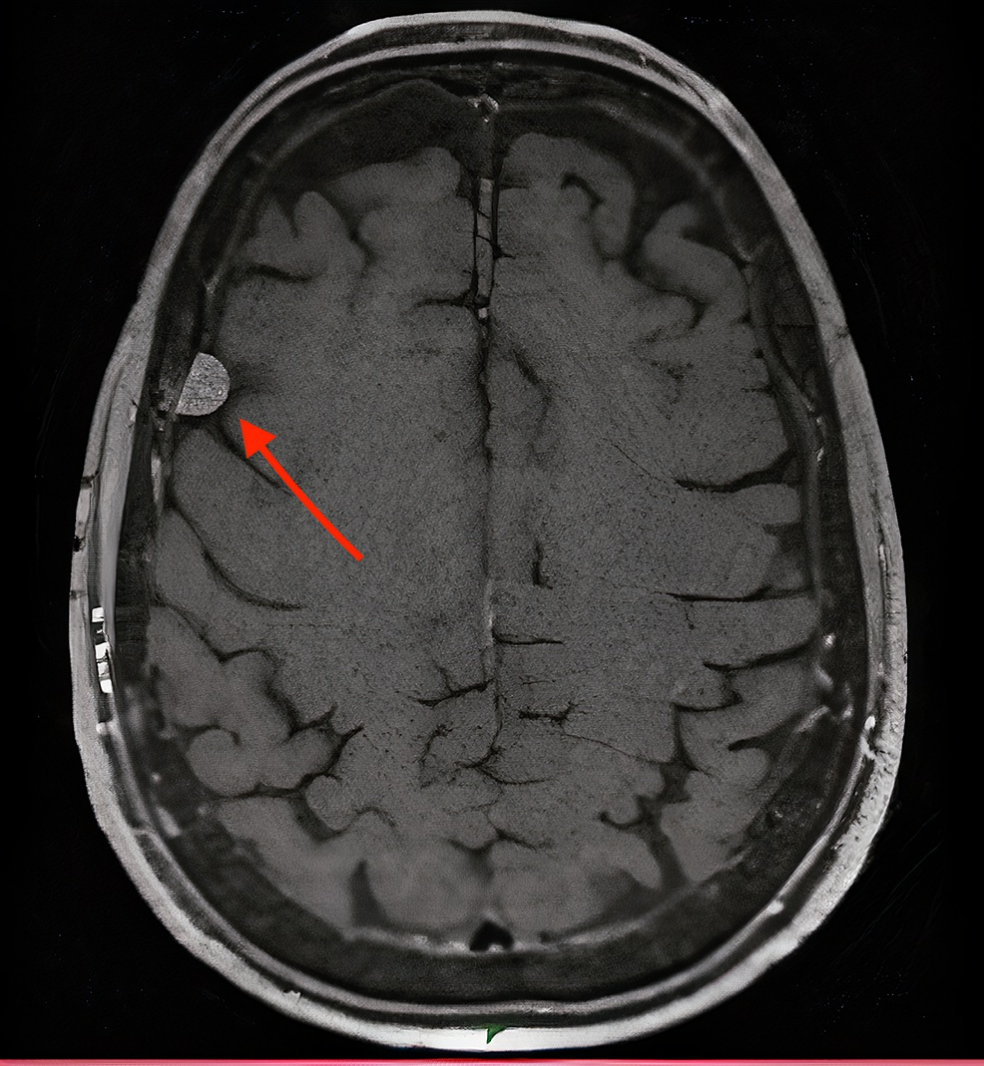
Gadolinium-enhanced T1-weighted magnetic resonance imaging Right frontal extra-axial formation: the size was 9 mm and there was no mass effect
